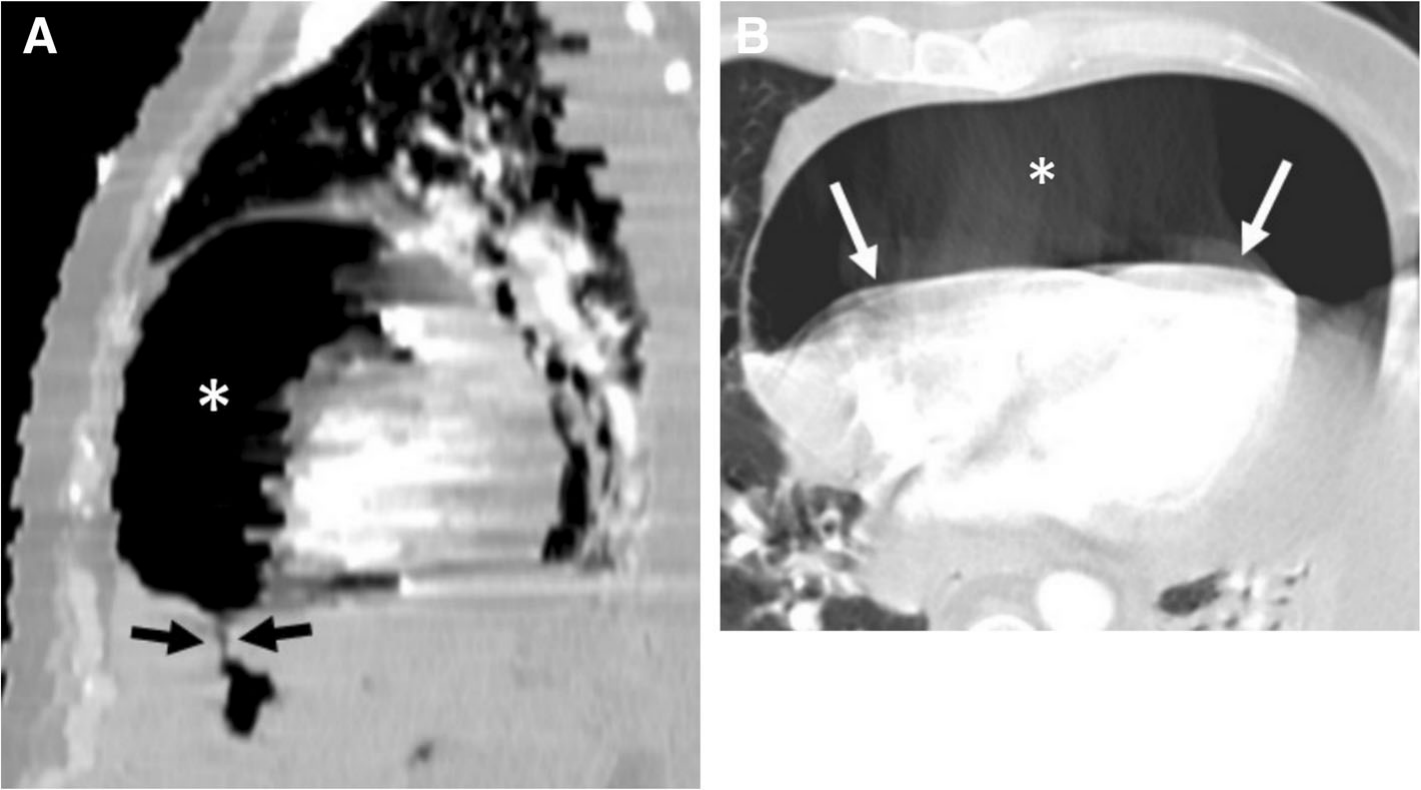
A 51-year-old man with pathologically proven gastric lymphoma developed sudden onset of dyspnea and cardiac arrhythmia. Contrast-enhanced CT in the sagittal (a) and axial (b) planes demonstrates a gastropericardial fistula (black arrows) complicated with massive pneumopericardium (asterisks). Significant compressive effect to the heart is also noted (white arrows)
